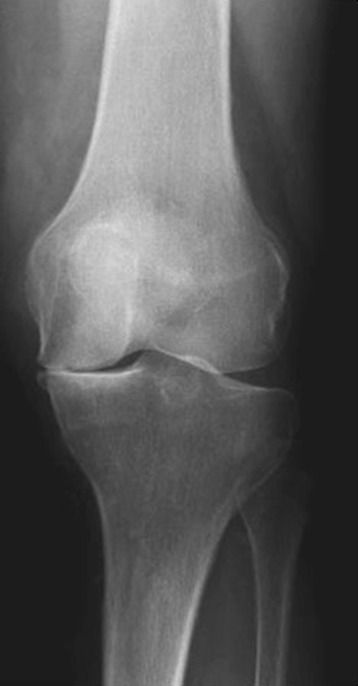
X-ray post subtotal meniscectomy. Forty two year old man: X-ray of a left knee joint 7 years after subtotal meniscectomy. It shows a lost joint line on the medial site and signs of osteoarthritis of the knee (published in (Zellner & Angele, 2017))
